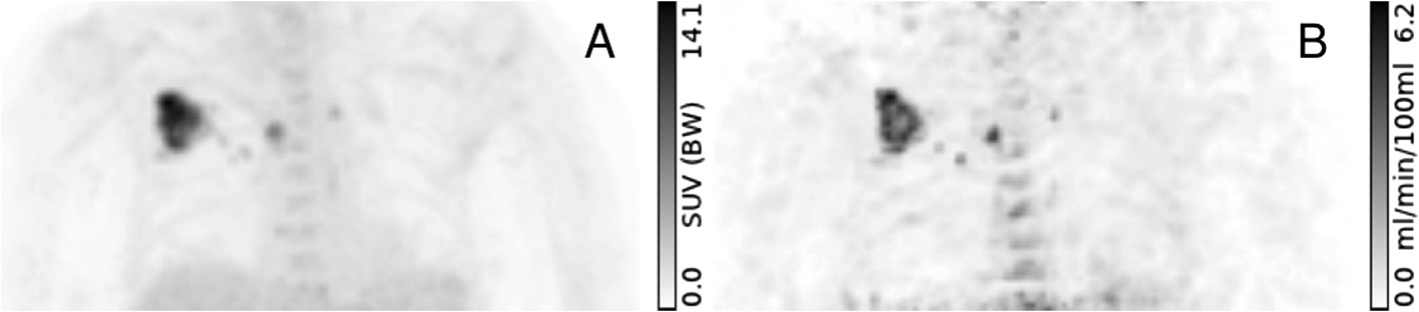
Comparison of parametric images of SUV and$K_{s}^{\left(c\right)}$.(A) FDG SUV 67 min. p.i. and (B) parametric $K_{s}^{\left(c\right)}$ map in a 63-year-old woman. The patient has a bronchial carcinoma of the right lung and lymph node metastases of the right hilar region and the mediastinum (coronal maximum projection). Note the different regional contrast and the used units in both images.
